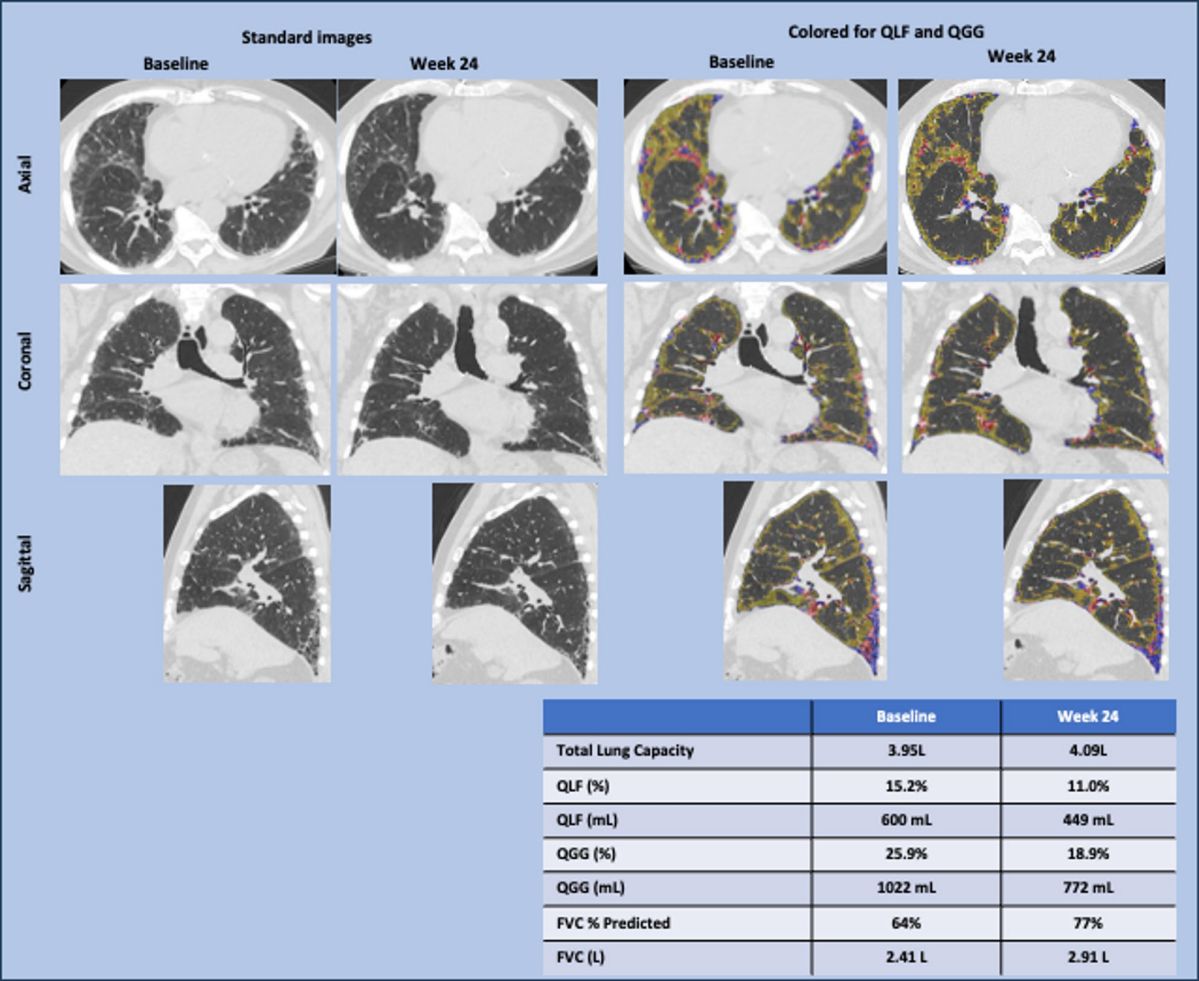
Baseline and week 24 images of a 100-mg bid patient showing improved QLF and FVC. bid = twice a day; FVC = forced vital capacity; HRCT = high-resonance computed tomography; QLF = quantitative lung fibrosis; QGG = quantitative ground glass. The images in the left two columns are the standard axial, coronal, and sagittal HRCT images for an individual patient treated with AP01 100 mg bid. The images in the right two columns are the overlaid qualitative results of the corresponding images. Blue dots indicate the results of QLF classification; yellow dots indicate results for QGG
